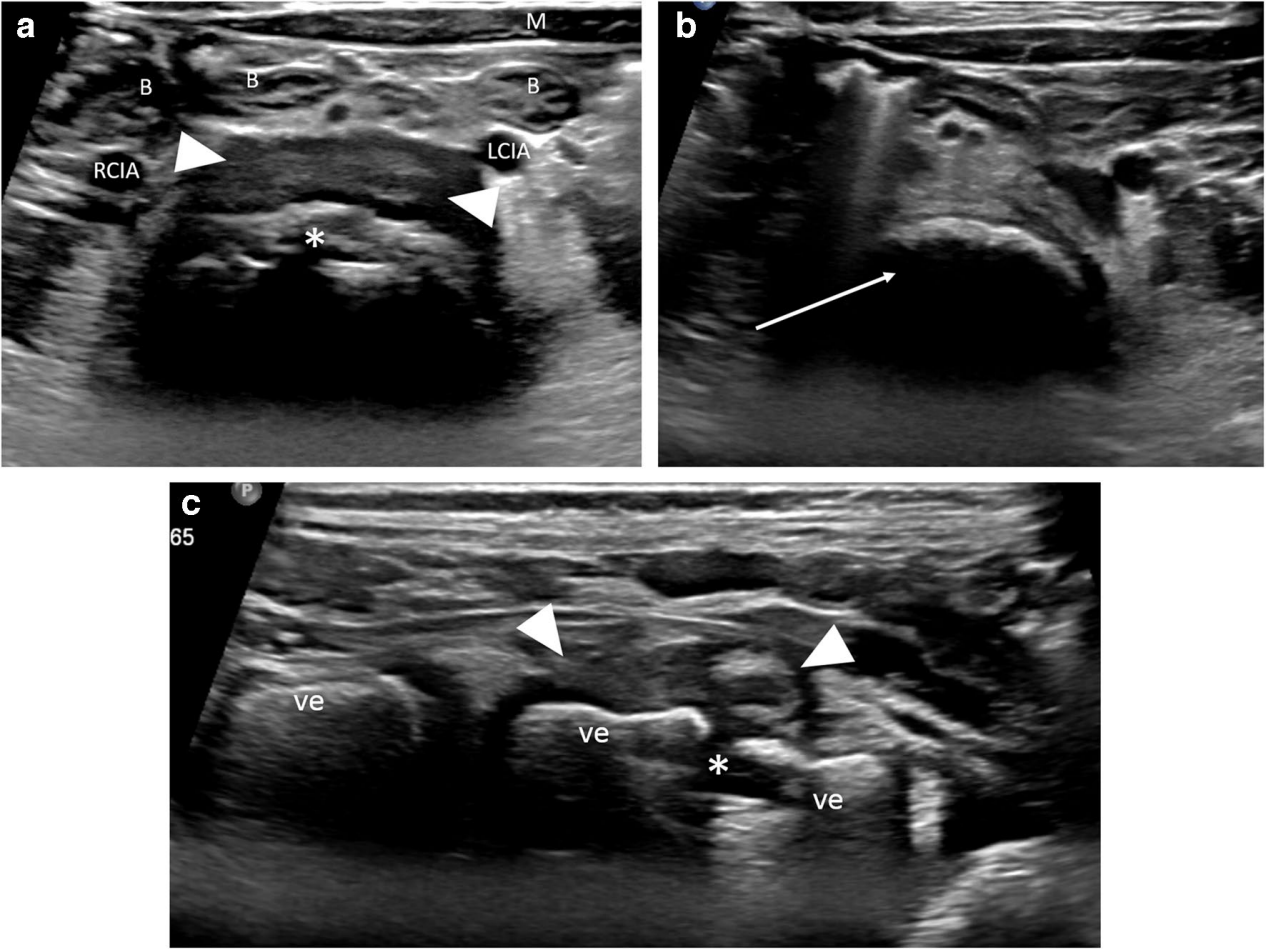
Ultrasound scan of the lower abdomen in a 21-month-old girl with spondylodiscitis. a Transverse image demonstrates a fragmented lumbar vertebral endplate (*asterisk*), with an anterior hypoechoic soft tissue mass (*arrowheads*). b Transverse image at a higher level shows a normal lumbar vertebral body (*arrow*) without endplate fragmentation, for comparison. c Longitudinal image shows an inhomogeneous anterior soft tissue mass (*arrowheads*) and narrowed disc space (*asterisk*). *B* bowel loops, *LCIA* left common iliac artery, *M* abdominal wall musculature, *RCIA* right common iliac artery, *ve* vertebral body
